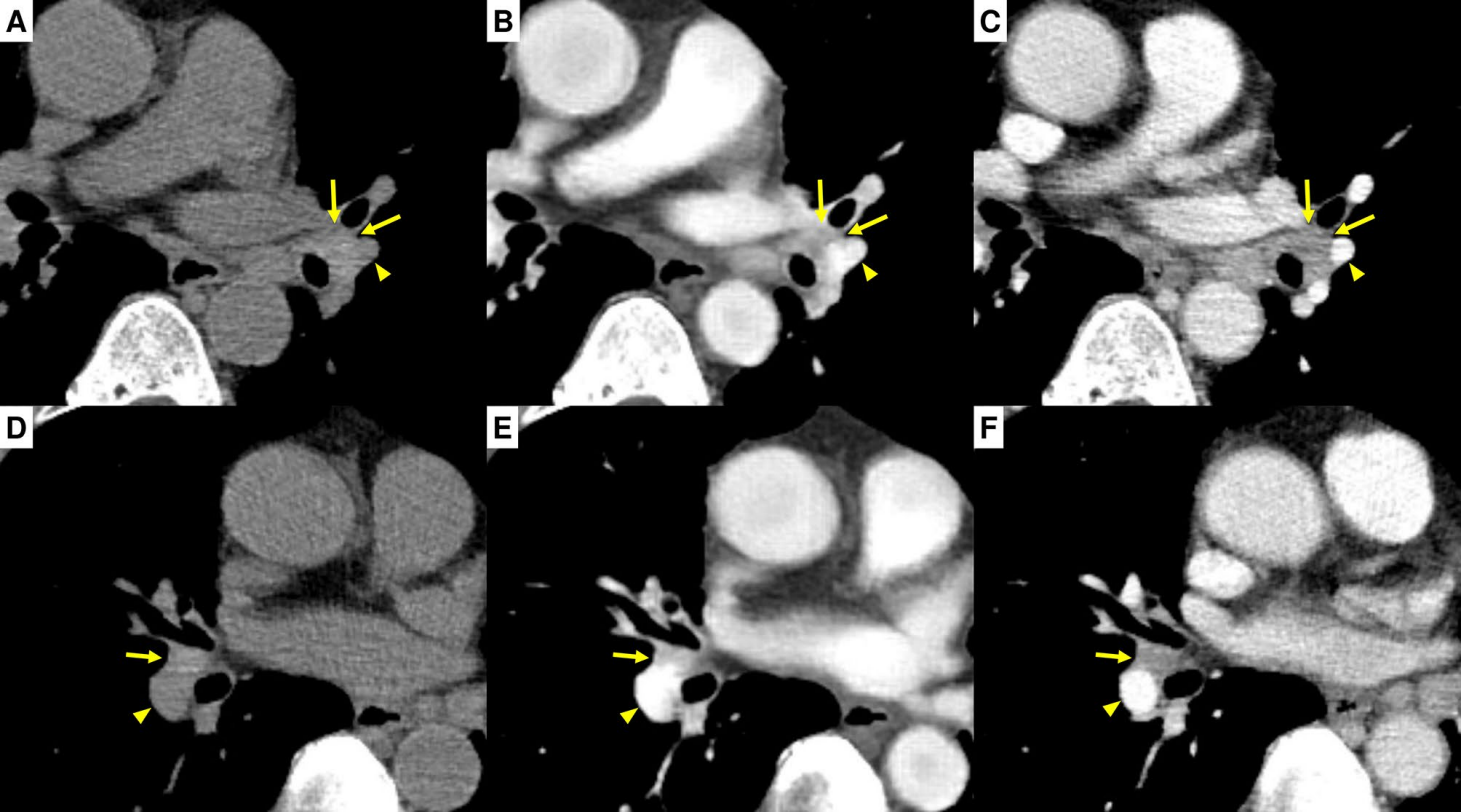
Images of a 78-year-old man with lung cancer and multiple mediastinal lymph node metastases from test set 2. Non-contrast CT (A, D), synthetic contrast-enhanced CT (B, E), and contrast-enhanced CT (C, F). Hilar lymph nodes (arrows), which are clearly visible on contrast-enhanced CT, are better distinguished from adjacent pulmonary vessels (arrowheads) on synthetic contrast-enhanced CT than on non-contrast CT.
